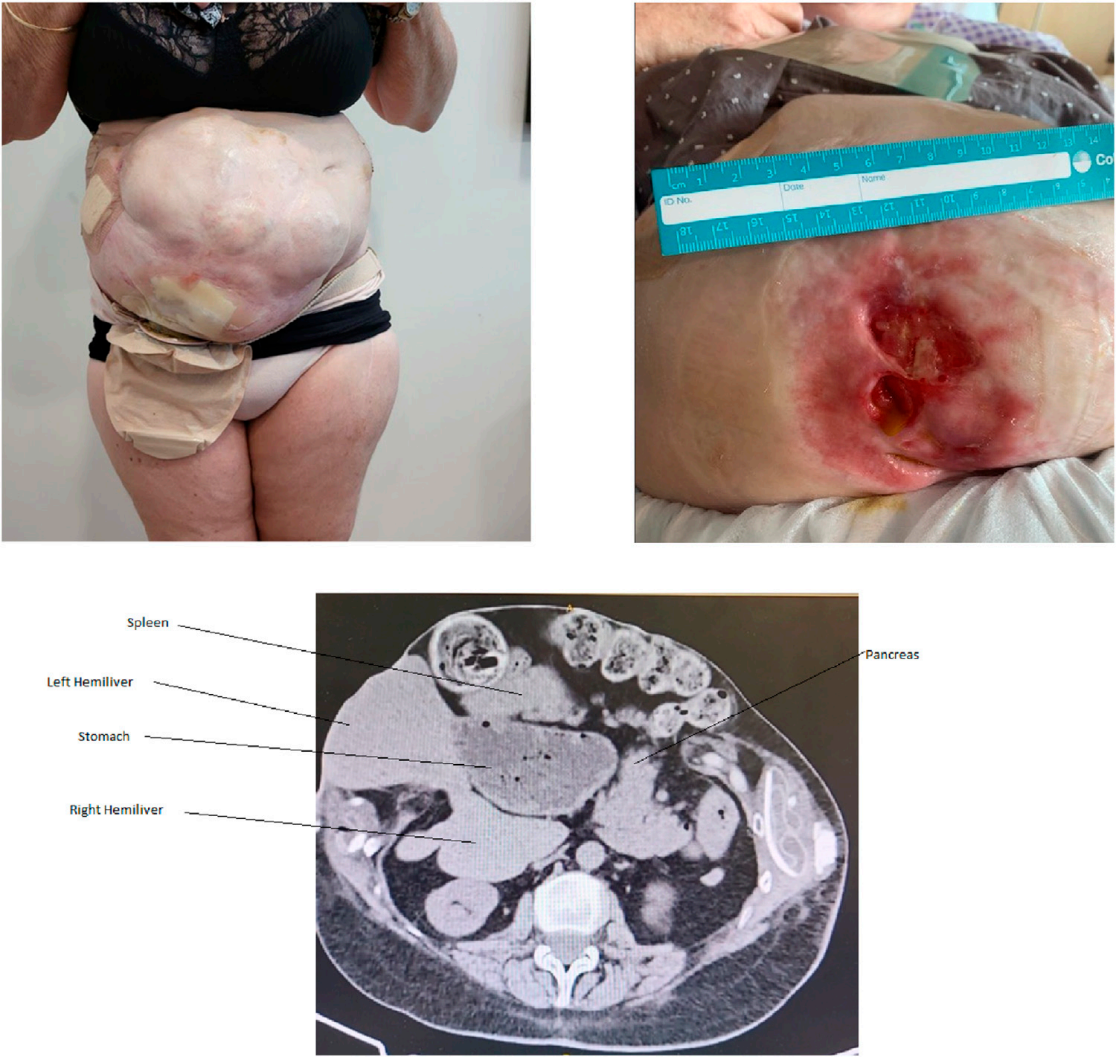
Patient at the time of most recent presentation with high volume output enteroatmospheric fistula involving the lower abdominal wall with severe skin maceration. In addition to 100% loss of domain, CT demonstrates malrotation of the viscera, with the foregut organs occupying a position in the right abdomen.
